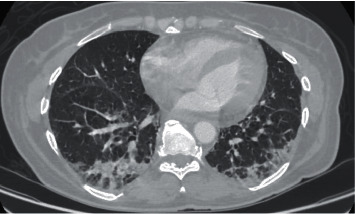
CT chest w/o contrast showing ground-glass opacities, bronchiectasis, and increased interstitial markings.
